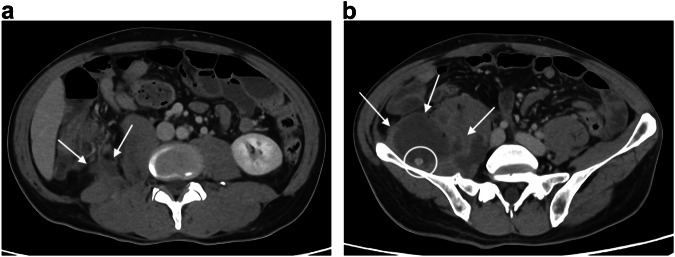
Complicated appendicitis. Axial portal phase CT (a, b) in a 76-year-old man shows a collection in the right psoas muscle (arrows), in which an appendicolith can be distinguished (circle), suggesting a complicated appendicitis with a psoas abscess
